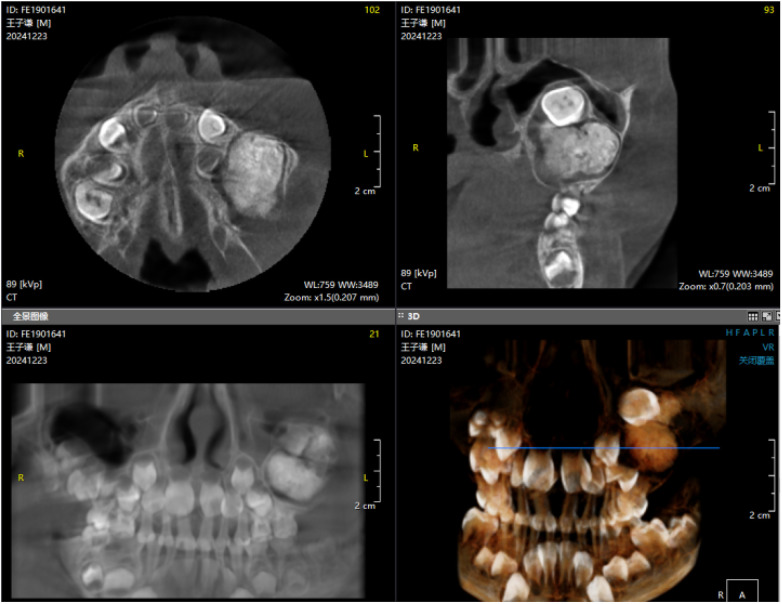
Preoperative CBCT image (1).
